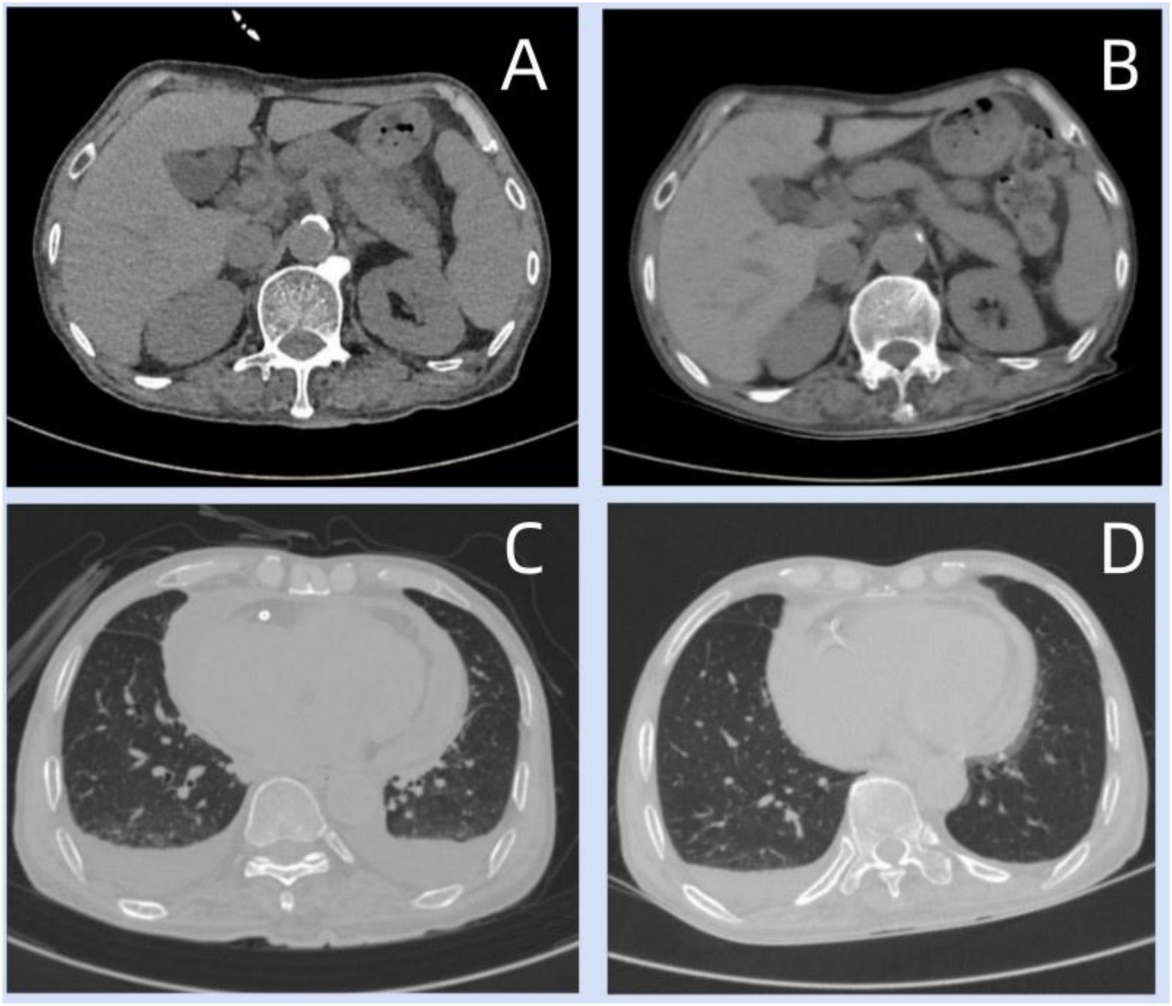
Before hormone treatment, the abdominal CT scan showed mild enlargement of the pancreas and a blurred fat plane around the pancreas (A); after hormone treatment, the CT scan showed that the pancreatic swelling had subsided and the surrounding exudation had largely absorbed. There was a small amount of inflammation in the lower basal segment of the right lung, and there was a small amount of effusion in both bilateral pleural cavities and pericardium (B); the three-dimensional reconstruction of the CT images before hormone treatment showed multiple small nodules in both lungs, with the maximum diameter approximately 0.4 centimeters, located in the posterior segment of the right lung lobe; there were patchy and cord-like high-density shadows in both lungs, with some edges being blurred (C); after hormone treatment, the patchy and cord-like density shadows in both lungs decreased, with some edges becoming blurred, showing a trend of improvement (D).
